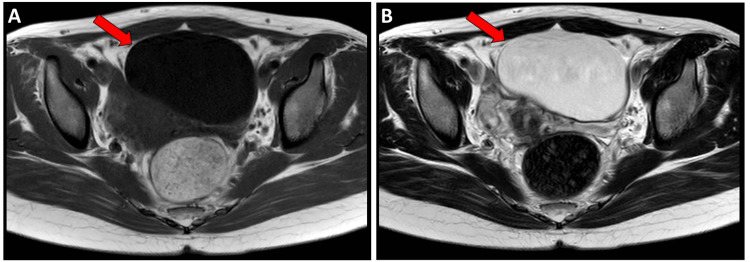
Pelvic magnetic resonance imaging findings. (A) T1-weighted image showing a unilocular cystic mass arising from the left ovary (arrow). (B) T2-weighted image demonstrating a high-intensity cystic lesion corresponding to the same ovarian mass (arrow).
